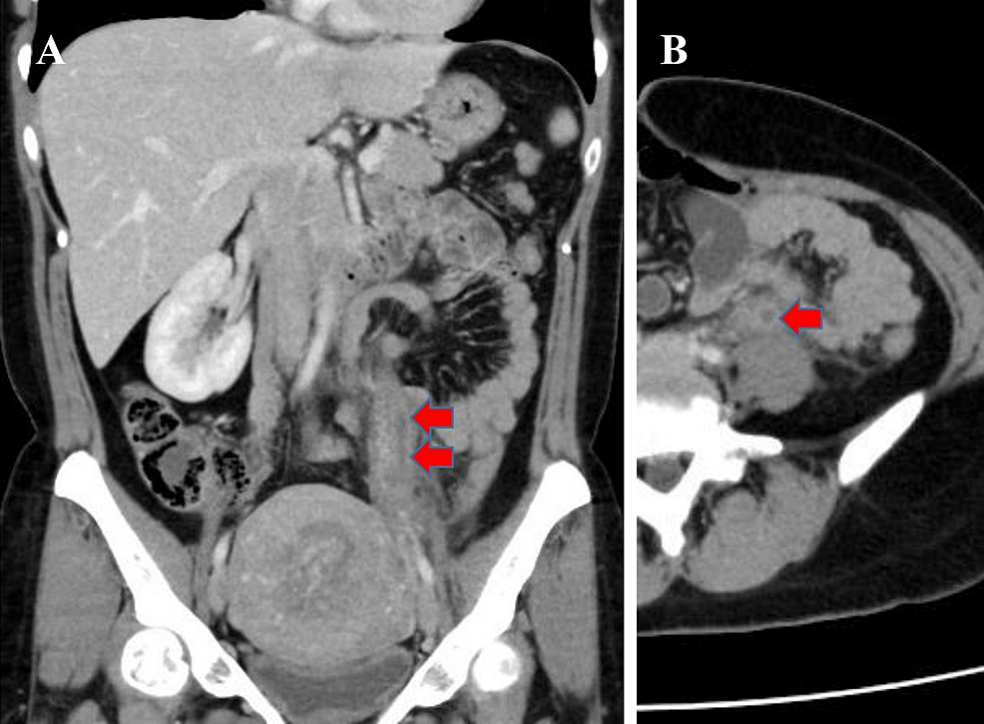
Contrast-enhanced computed tomography of the abdomen showing a thrombus of the left ovarian vein (arrow).
